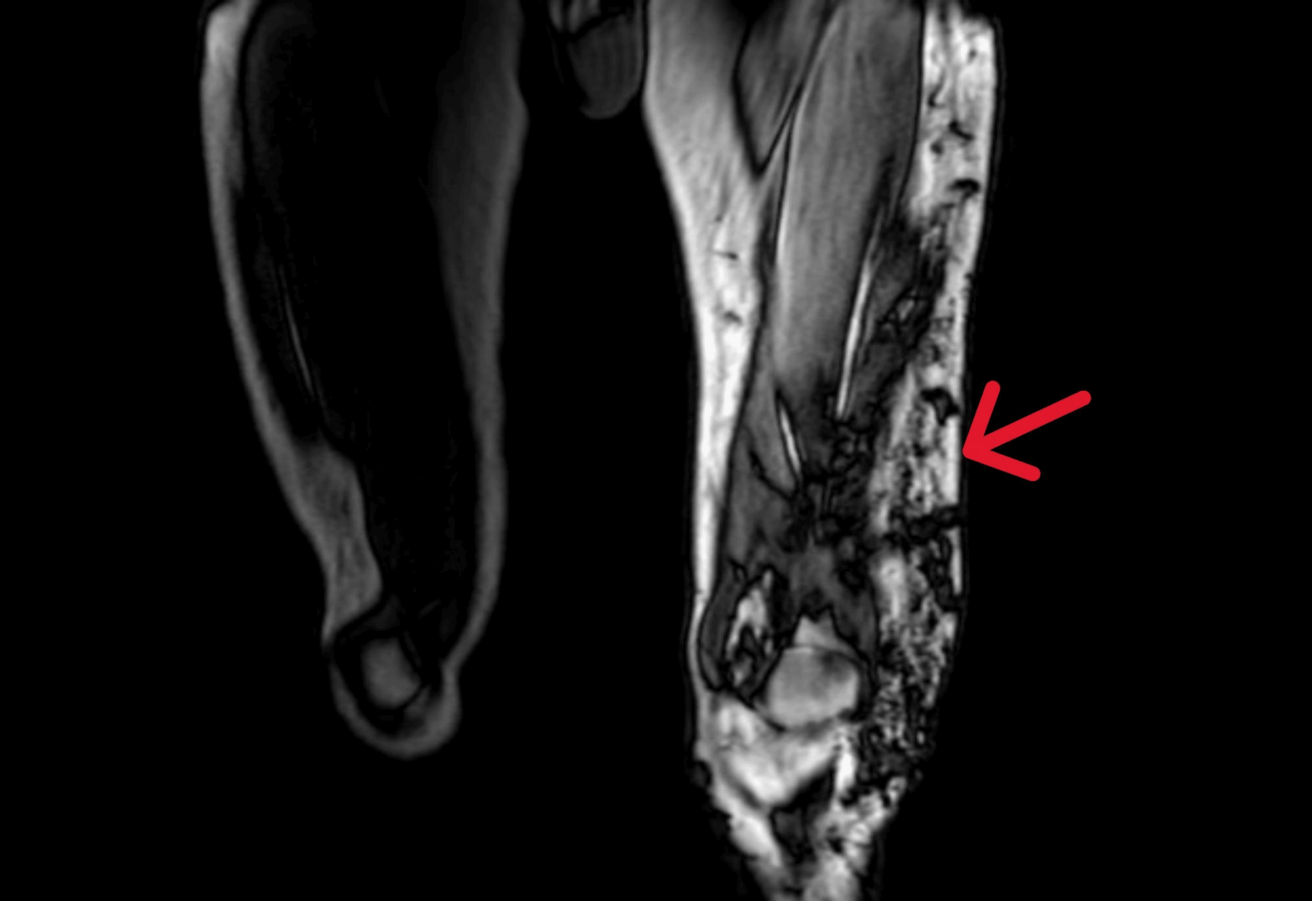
MRI of the left lower limb revealing numerous superficial and deep varicose veins with further superficial draining varicosities. There is diffuse left lower limb enlargement with dilated lymphatics.
